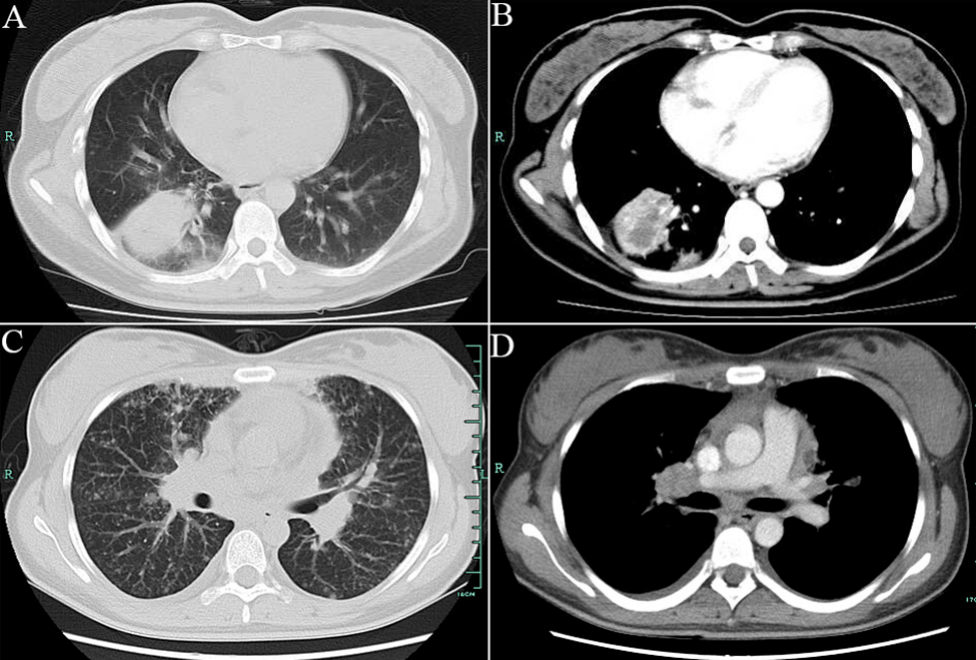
Thoracic CT findings of representative NSCLC patients with EML4-ALK gene fusions. A, B: mass with no GGO; C: multiple nodules; D: multifocal lymphadenopathy.
